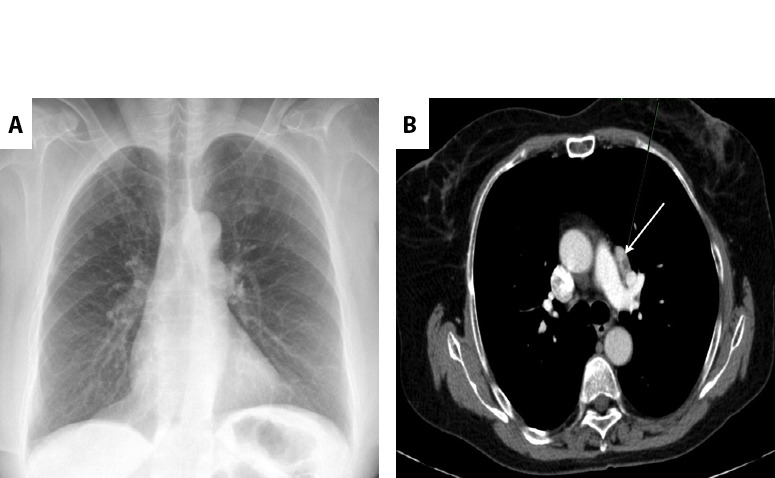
Chest X-ray (A) and computed tomography scan (B) revealing hilar fullness.
